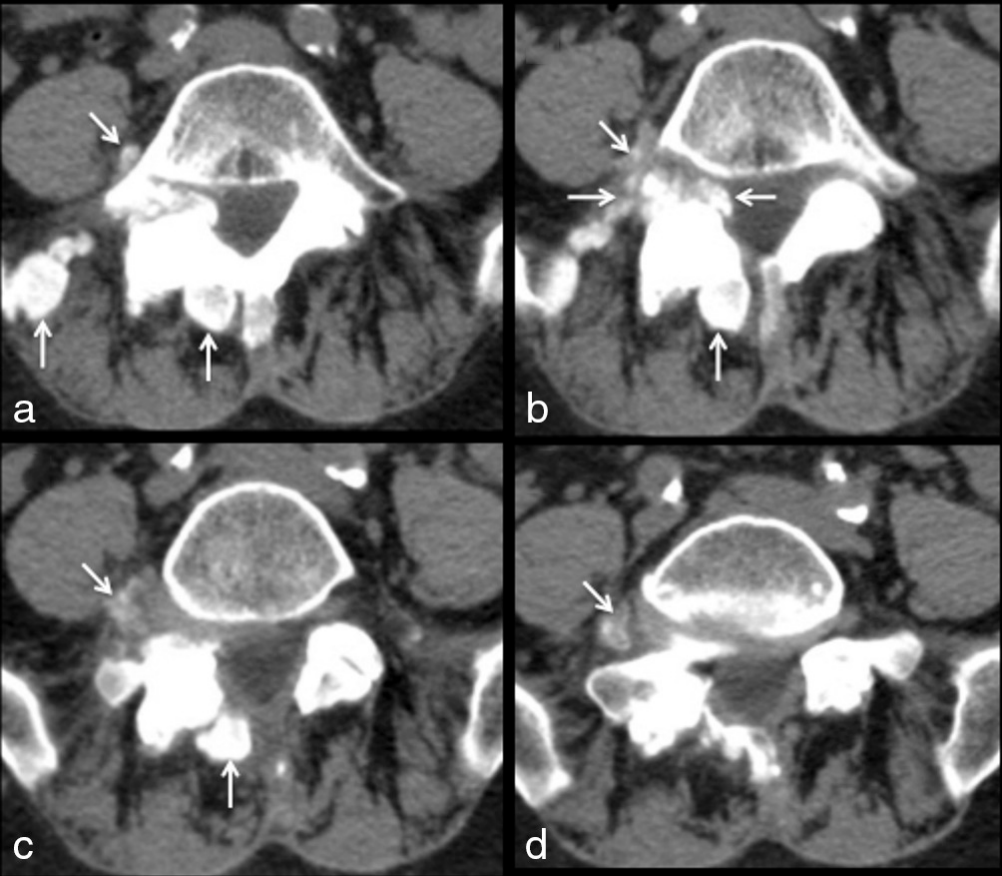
CT scan of the lumbar spine. Axial CT images with soft tissue window (a–d) showing calcified mass-like lesions in the paraspinal soft tissues at the same locations described on MRI (white arrows). The symptomatic lesions involve the right L5–S1 neuroforamen and have extraforaminal extension. The lesion located at the right paracentral epidural space is not well seen on CT owing to its lower attenuation compared with the other lesions.
